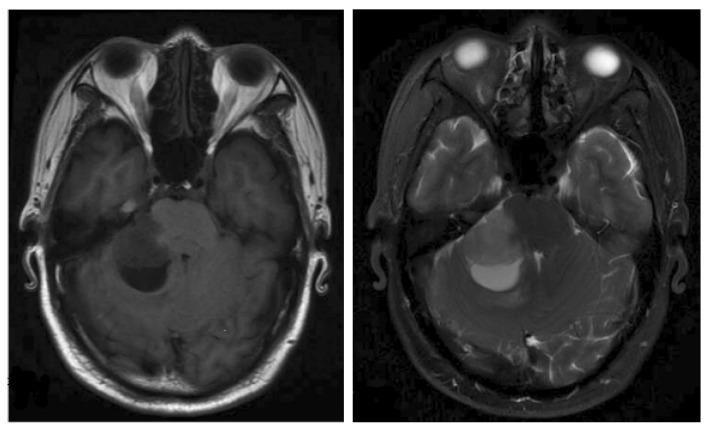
A large abnormal signal lesion was visible in the right cerebellar hemisphere with a size of ∼2.7×3.4 cm and an obscure boundary. T1-weighted imaging (T1WI; left panel) demonstrated low signals while T2-weighted imaging (T2WI; right panel) demonstrated a marginally higher signal intensity. Enhancement scanning revealed an inhomogeneous enhancement. A long T1 and long T2 signal revealed a cystic lesion and a patchy edema zone was visible in the peripheral lesion. The pontine was shifted marginally to the left, while the fourth ventricle was deformed and compressed.
